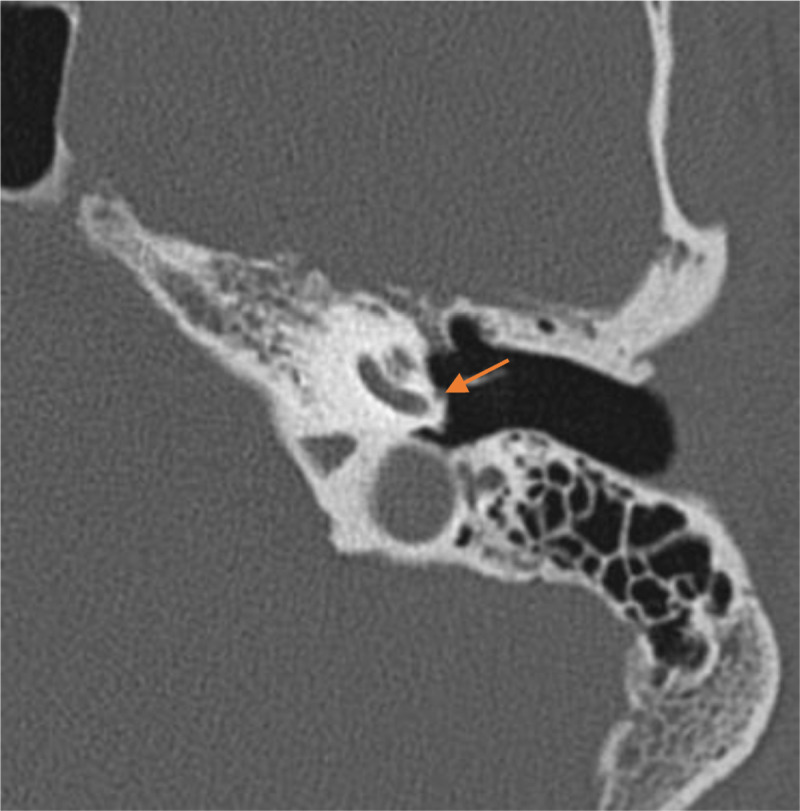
Axial CT scan showing the PSA on the cochlear promontory.
